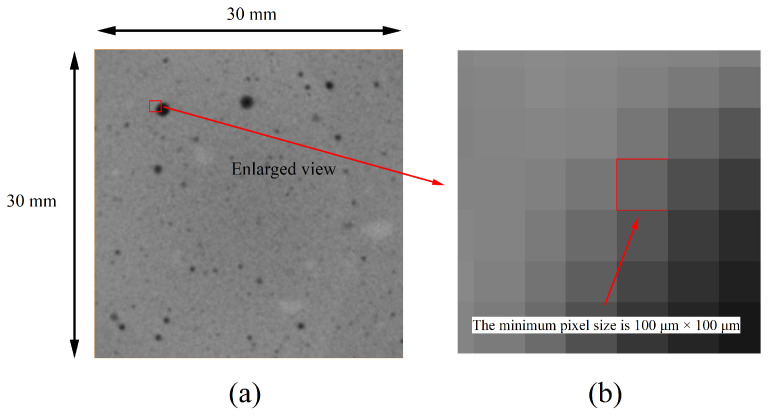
CT image of (a) original image and (b) enlarged image.
